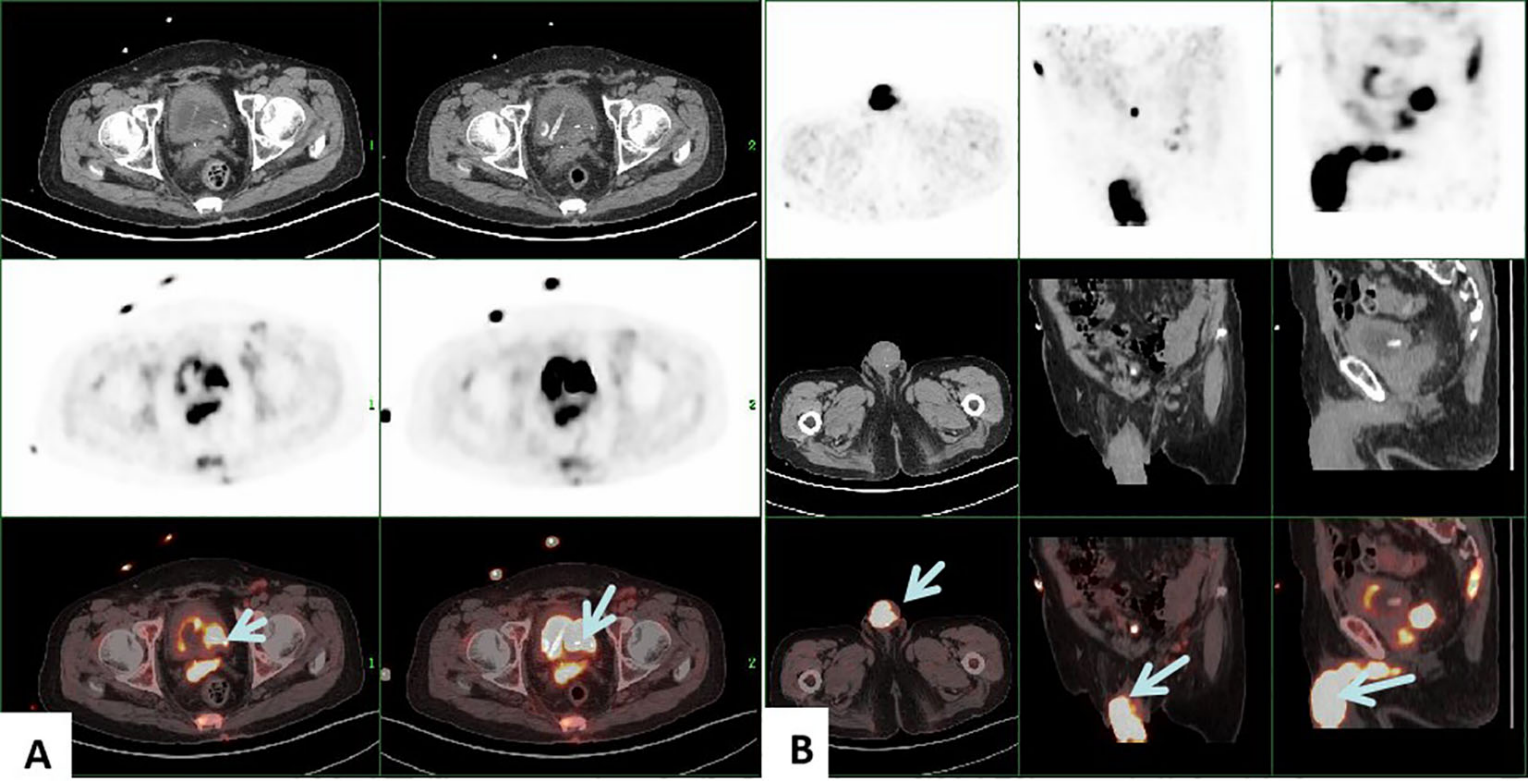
PET/CT revealed that the higher uptake of prostate gland (SUVmax 15.3) (A) and penile lesions (SUVmax 18.7) (B), indicating local recurrence and penile metastasis from prostatic cancer.
